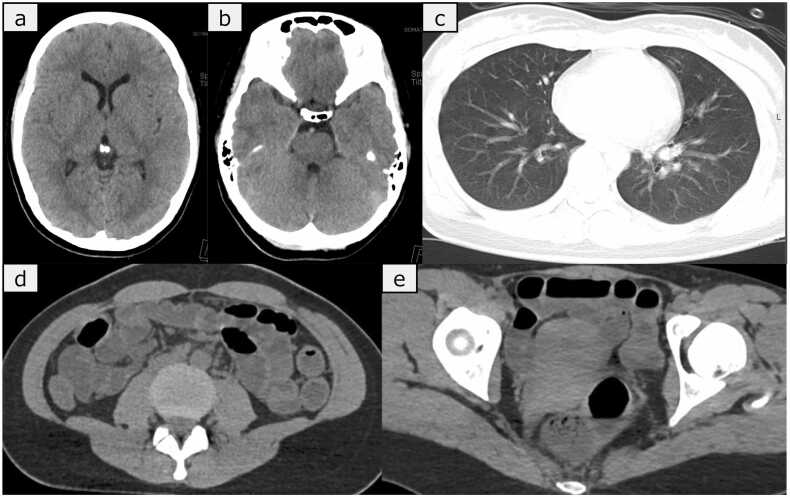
Non-contrast CT on admission. CT imaging shows no intracranial hemorrhage or hematoma in the head (a, b) and no abnormalities in the lung fields (c). Abdominal imaging reveals dilation of the small intestine with fluid accumulation but no evidence of obstruction or ascites (d) and no abnormalities in the ovaries (e).
